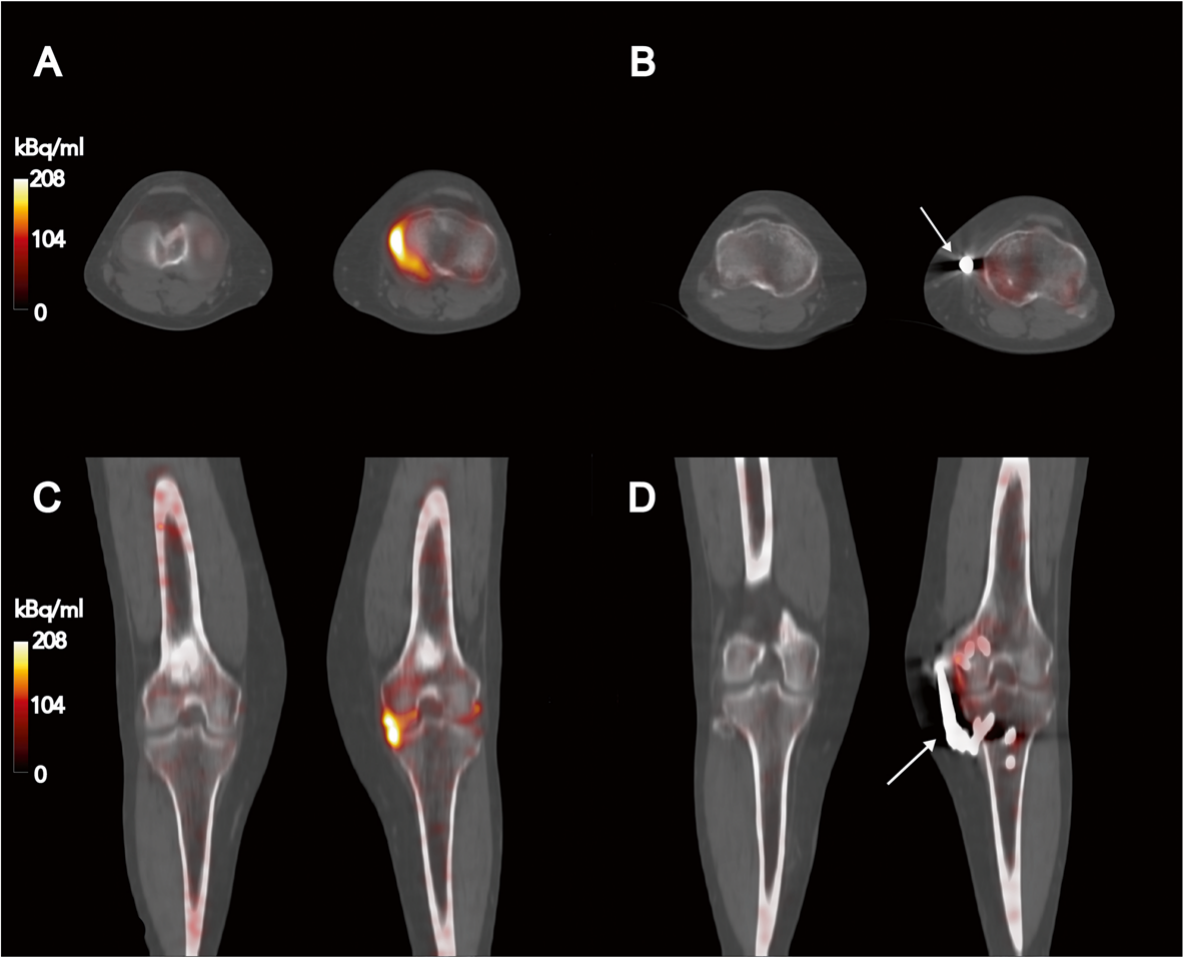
a, c Pre-OP SPECT-CT images from a patient (f, 46 year, SPECT-CT: 5 months pre-implantation) with beginning knee OA and b, d corresponding post-op imaging (14 months post-implantation) exemplifying the effect from the examined load absorber (b, d: metal artifact labeled by arrow). Patient showing a significant decrease in bone turnover after implantation at the level of the left tibia plateau (AC_max, pre_ = 309.2 kBq/ml, [SUV_max_ = 77.5] and AC_max, post_ = 99.0 kBq/ml, [SUV_max_ = 31.7]). Accumulation patterns from bone turnover (pre/post) were windowed identically
